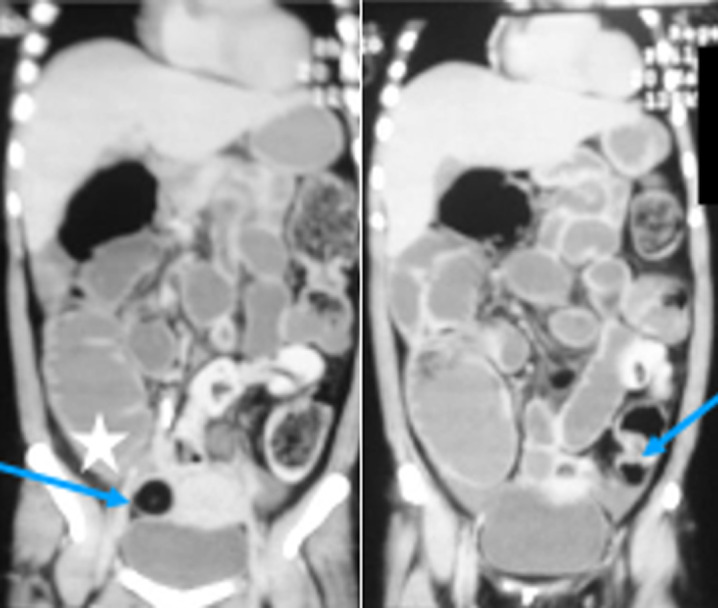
coronal views of CT scan reveal dilated large intestine up to caecum (asterix) with no zone of transition, normal small bowel caliber, and no ascites or pneumoperitoneum. Incidentally, the patient was incidentally found to have bilateral adnexal dermoid cysts (blue arrows)
